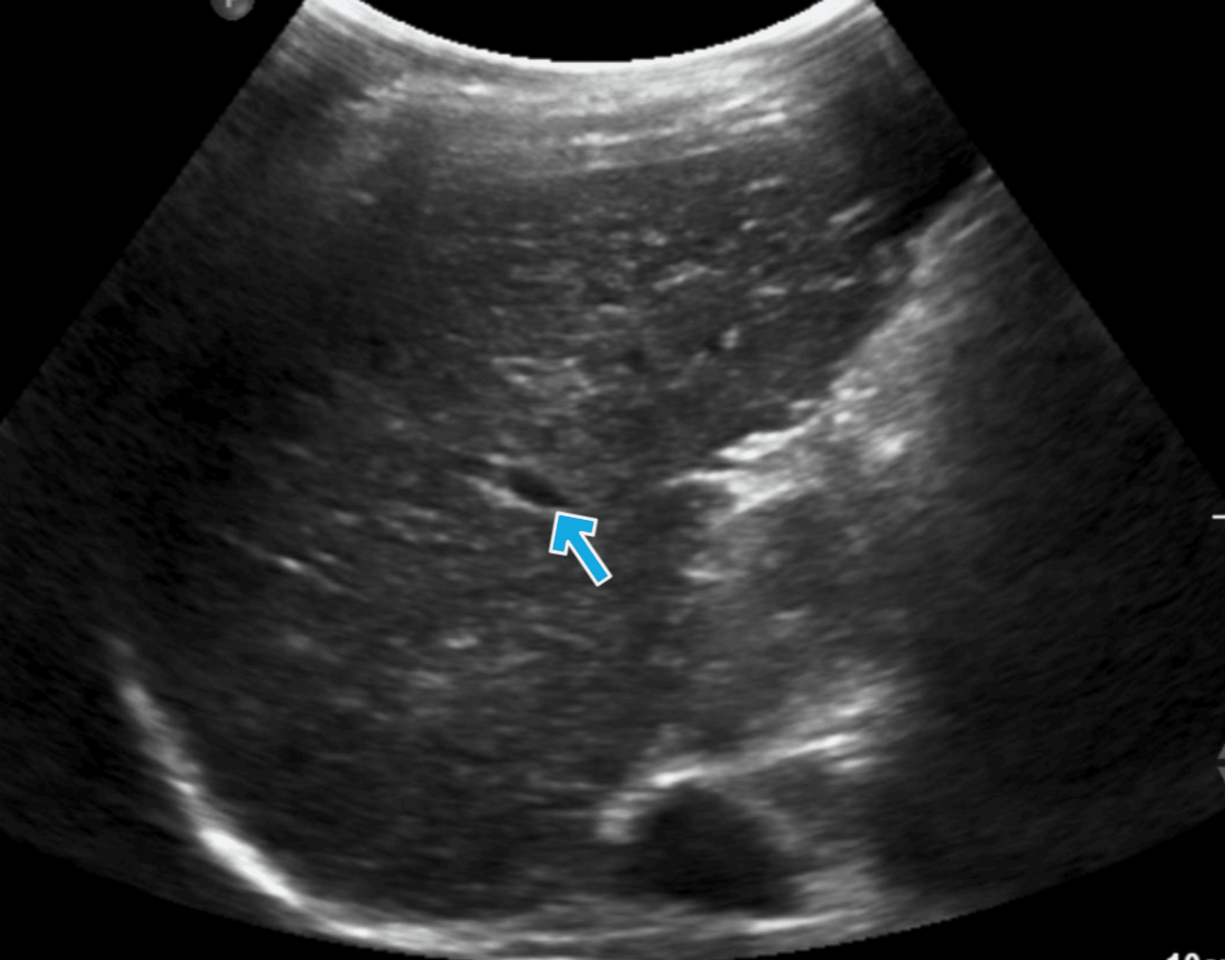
Ultrasonography of the abdomen of the middle sibling The image shows chronic liver disease with altered liver echoes. The arrow indicates the altered echo texture with regenerating nodules.
